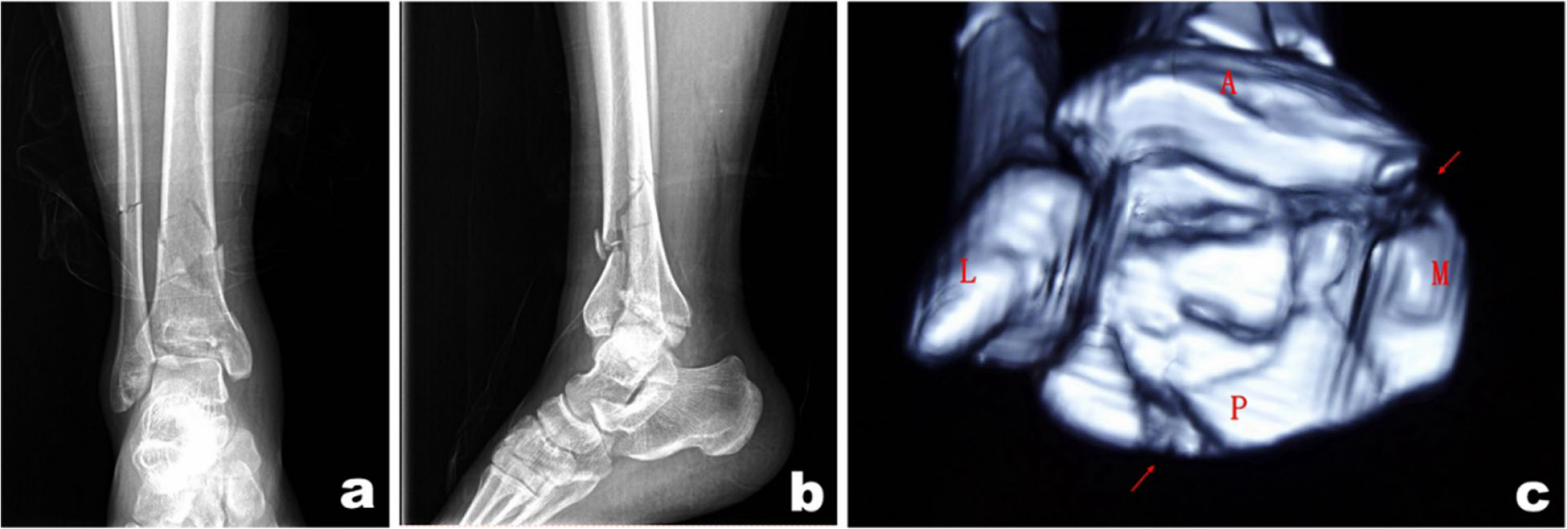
Male, 37 years old, right tibia pilon fracture caused by fall injury. Preoperative X-ray (a, b) and CT scan (c) showed collapse of the articular surface, the posterior malleolar fragment did not involve the tarsal tunnel area, and the die-punch fragment was slightly anterior and medial
